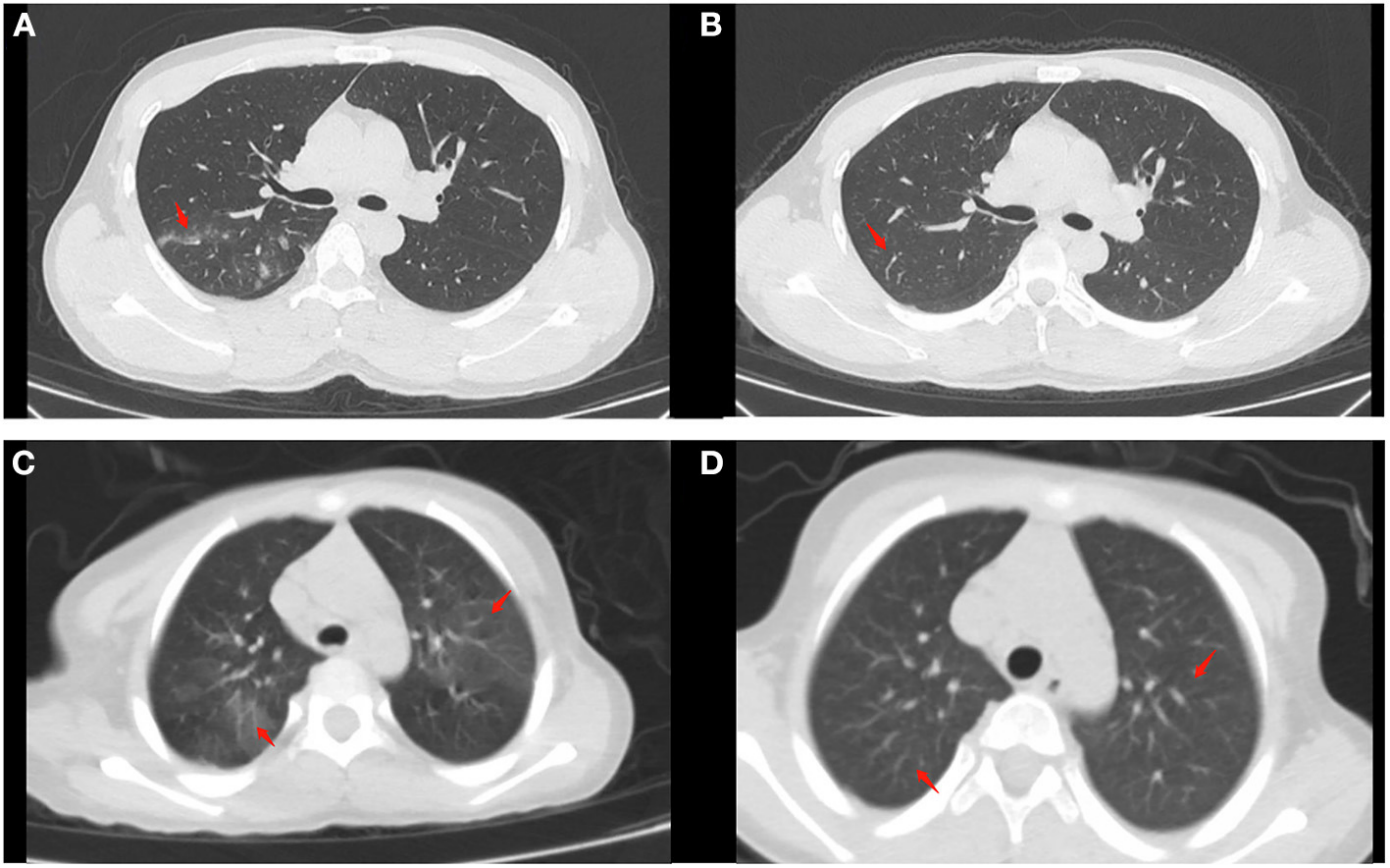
Chest CT findings of moderate cases before and after treatment (as arrow points). (A) Patchy high-density shadow can be seen in the posterior segment of the upper lobe of the right lung before treatment in naïve patients. (B) The high-density shadow subsided after treatment in naïve patients. (C) Patchy high-density shadow and blurred edges can be seen in the upper lobes of both lungs in a refractory patient. (D) No significant abnormalities can be seen after treatment in the refractory patient.
